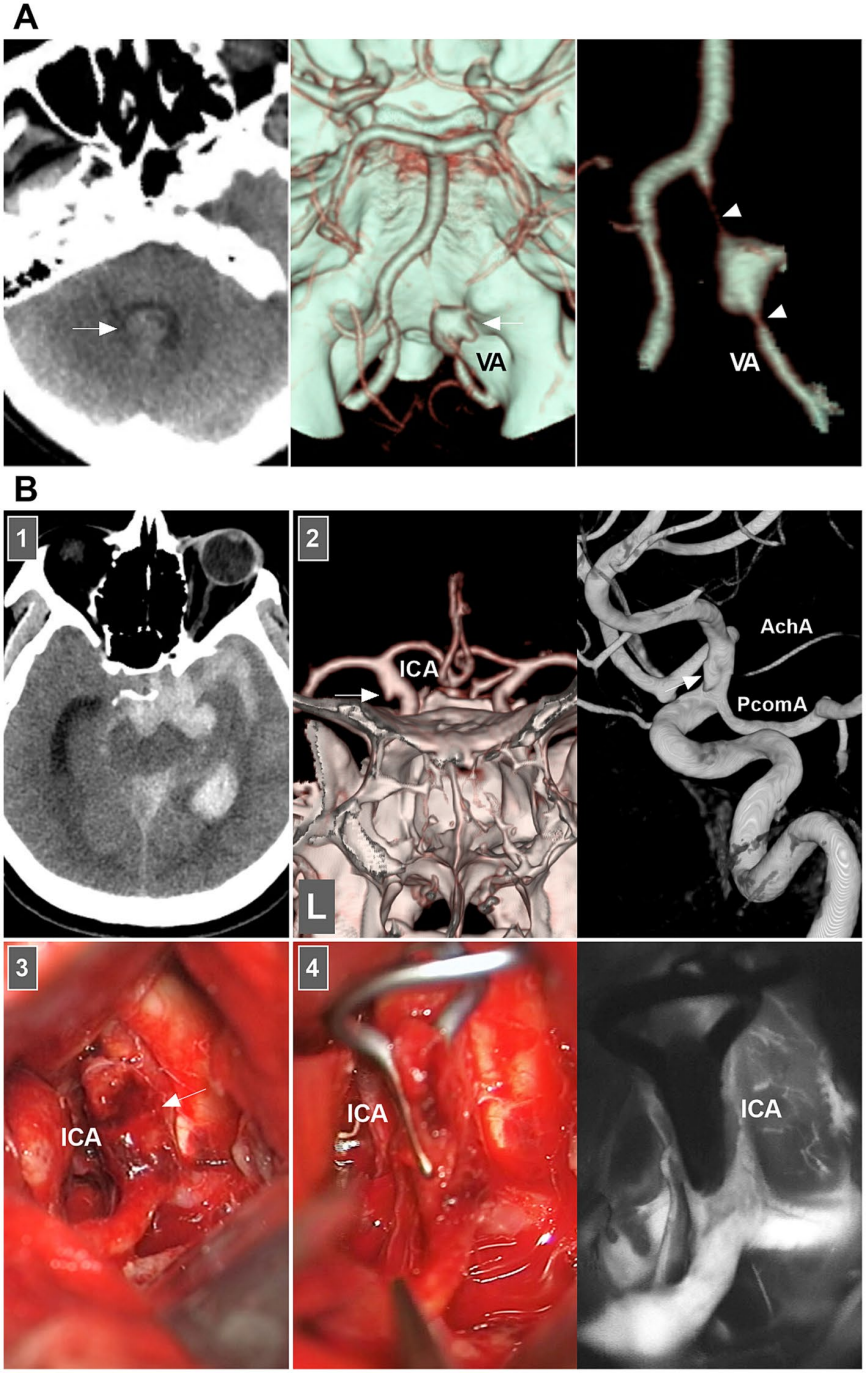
IAD appearance on angiography and open surgery. (A) Left panel: CT showing the hemorrhage in the fourth ventricle (arrow). Middle and right panels: CT images showing a typical VA dissection (arrow in middle panel) presenting with dilatation with stenosis (arrowheads in right panel). (B) Panel 1: CT showing subarachnoid hemorrhage into temporal horn of lateral ventricle. Panel 2: CTA (left panel) and DSA (right panel) images showing a protrusion (arrows) on the left supraclinoid ICA above the PcomA and opposite to the AchA, indicating that the lesion was a dissection or blood blister-like aneurysm. Panel 3: Intraoperative image showing that supraclinoid ICA had subadventitia hemorrhage (arrow), confirming to the dissection not a blood blister-like aneurysm. Panel 4: Intraoperative image (left panel) showing the clipping was performed, intraoperative fluoroscopy (right panel) showing that intracranial ICA was patent. AchA, anterior choroidal artery; CT, computed tomography; CTA, CT angiography; DSA, digital subtraction angiography; IAD, intracranial arterial dissection; ICA, internal carotid artery; PcomA, posterior communicating artery; VA, vertebral artery.
